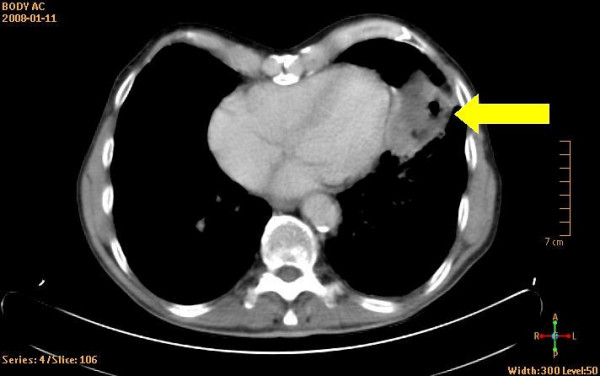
Display of CT-image. The arrow indicates the non-small cell lung cancer.
